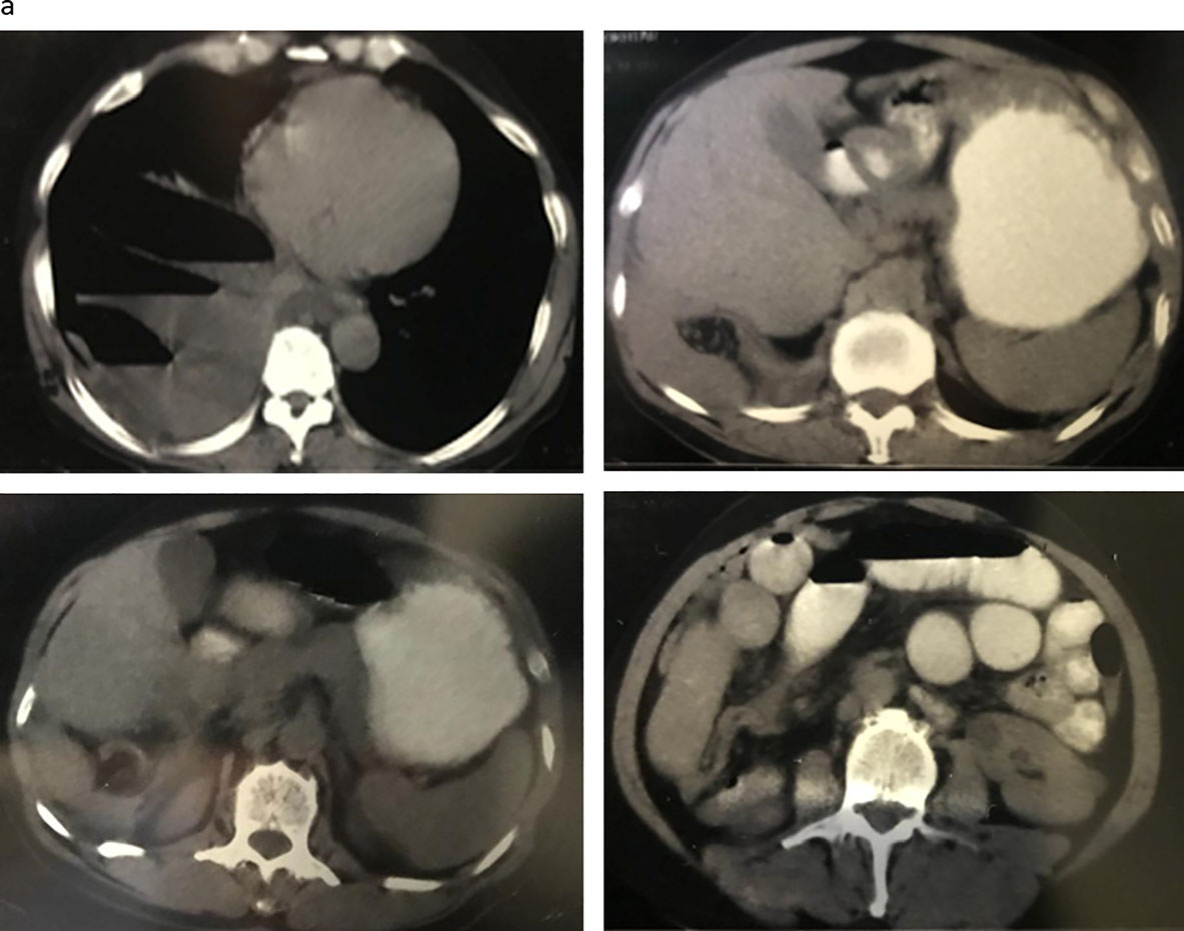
CT scan of patient showing air-fluid level in the intestine, presence of intestinal loop behind the liver through right hemidiaphragm into the right hemithorax
